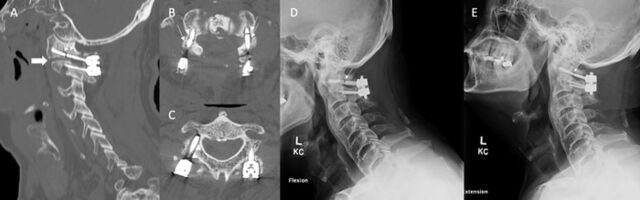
Noncontrast computed tomography (A) sagittal view of the craniovertebral junction at the plane of the left facet demonstrates a fractured C1 lateral mass screw (small arrows) and a C2 pars articularis screw with the tip in the region of the C2 transverse foramen. Failed arthrodesis is noted at the atlantoaxial articulation (block arrow), which was consistent contralaterally. Axial sequences at the (B) C1 and (C) C2 levels show bilateral fractured lateral mass screws (arrows) and pseudoarthrosis of the right pars screw (white arrowheads on medial aspect). Lateral (D) flexion and (E) extension dynamic plain films of the cervical spine show movement of the fractured lateral mass screws (arrows).
